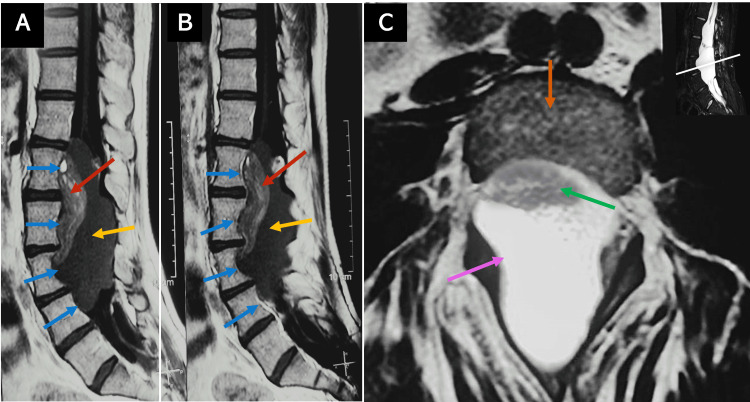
Preoperative MRI (A, B) Preoperative sagittal T1 MRI of the lumbar spine showing a heterogeneous lumbar tumor lesion with well-defined borders that modifies the morphology of the L1-L4 vertebral bodies (blue arrows). It presents a ventral hyperintense component, a head tumor with fat tissue (red arrow), and a dorsal hypointense component, a body tumor (yellow arrow). (C) Axial T2 MRI at L3 shows the intradural body component of the tumor occupying the entire diameter of the spinal canal (pink arrow). The head of the teratoma is indicated by the green arrow. The vertebral body corresponds to the orange arrow. MRI: Magnetic resonance imaging, L: lumbar spinal level
